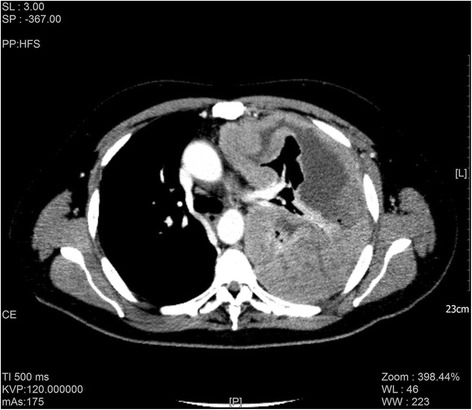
CT image revealed irregular circumferential pleural thickening with nodular soft tissue masses, involving the mediastinal and diaphragmatic pleura, and extending into the fissures, with reduction of right lung volume
